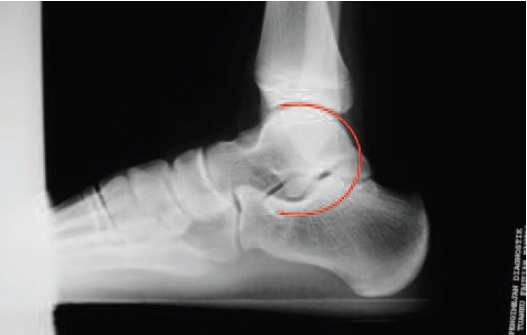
Subtle C sign on right ankle lateral view X-ray.
